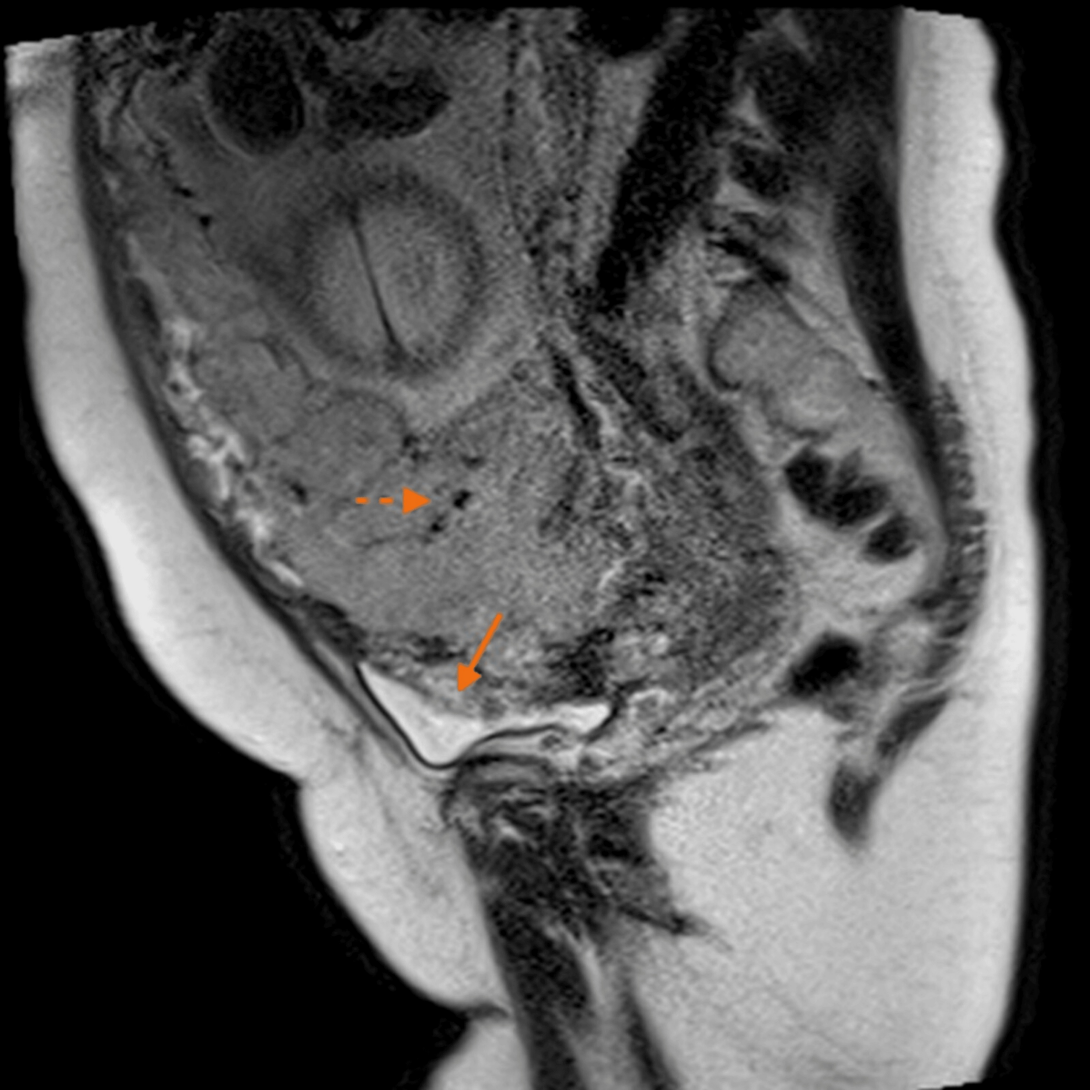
Magnetic resonance imaging in the sagittal plane in T2 sequence without fat saturation. We observe the loss of the hypointense bladder wall secondary to placental invasion (arrow). Flow voids (dashed arrow) are observed in relation to anomalous intraplacental vessels.
